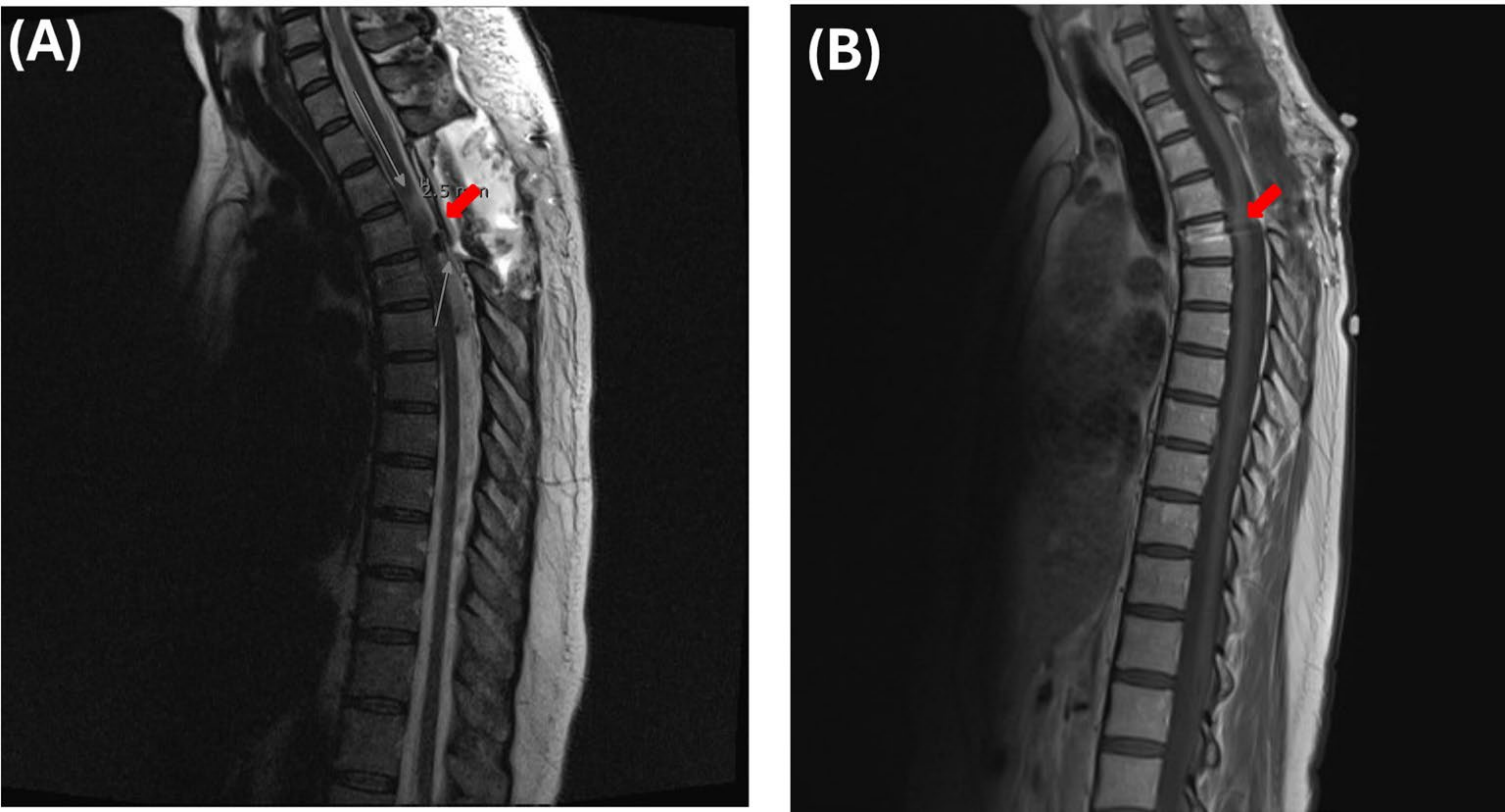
(A) Post‐operative, and (B) most recent follow‐up MRI of the thoracic spine with and without contrast, demonstrating no residual lesion at the T3–T4 level (Red Arrows).
